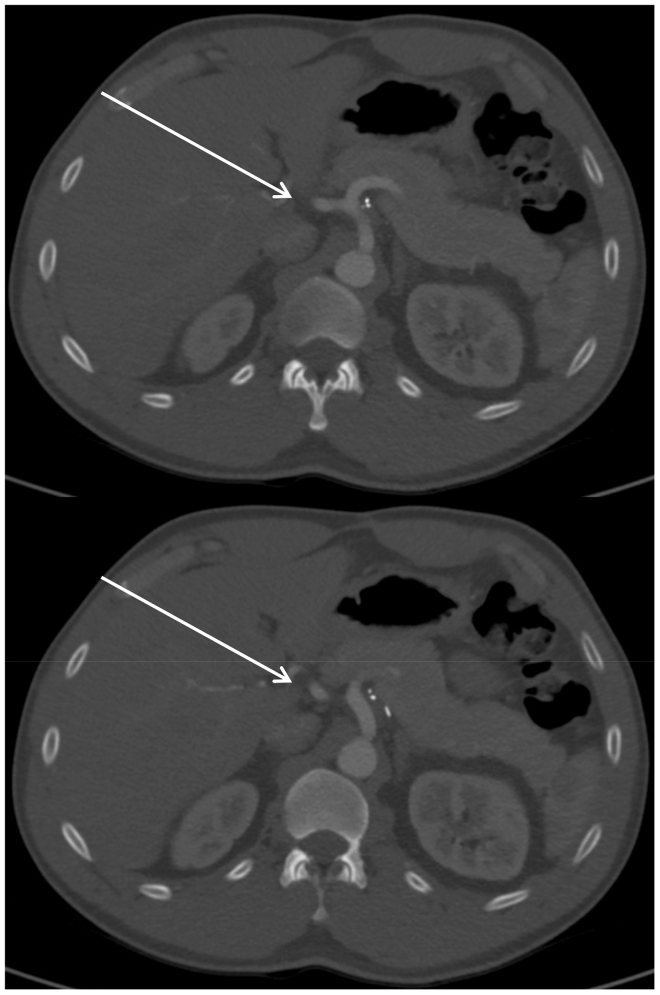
Computed tomography angiography (CTA) performed 9 months postoperatively with showing well-enhanced interposition graft and patency (*arrow*).
